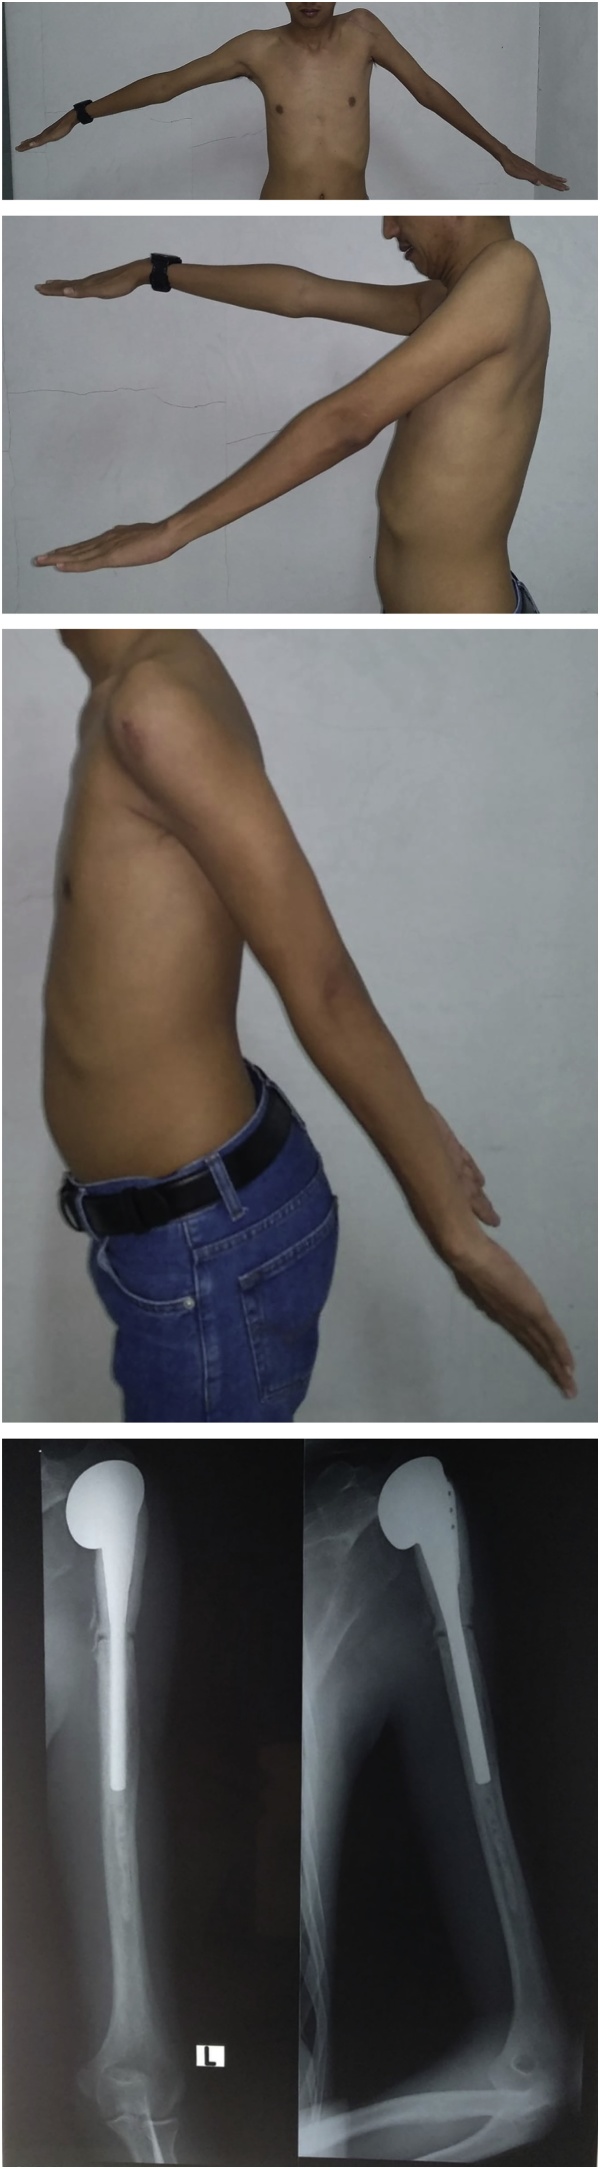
Clinical Outcome and post operative radiograph at 6 months post operative.
